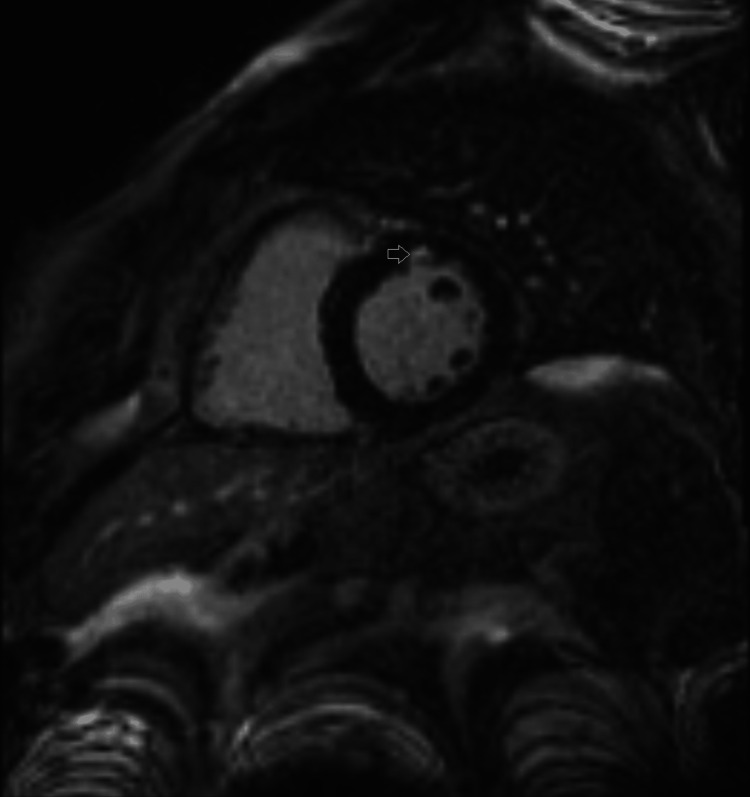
Cardiac MRI showing a focal area of late gadolinium enhancement in the mid-wall (non-ischemic pattern) located at the basal segment of the anterior wall
